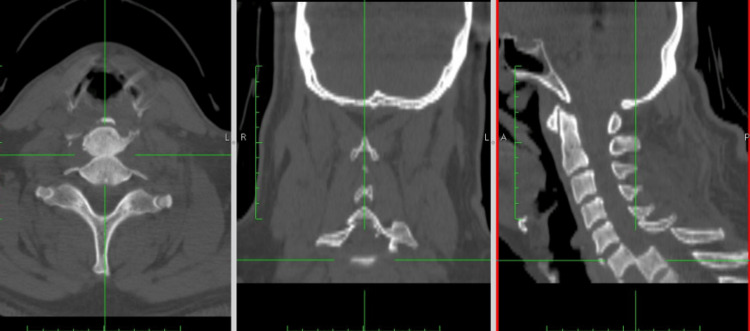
Case 2 CT scan of the C6-C7 injury in axial, coronal, and sagittal views.
